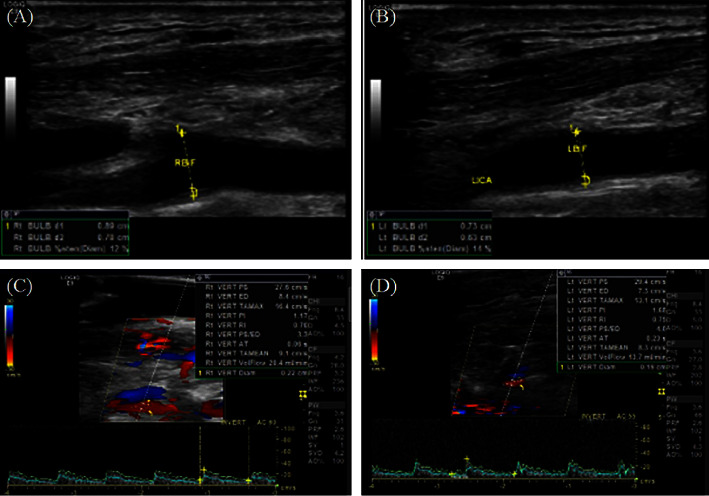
Neck ultrasound for carotid artery study. The data showed mild atherosclerosis (< 15%) of bilateral carotid bifurcations and bilateral small-diameter vertebral arteries, with reduced flow volume (34 ml/min). (a) Right carotid bifurcation. (b) Left carotid bifurcation. (c) Right vertebral artery. (d) Left vertebral artery.
